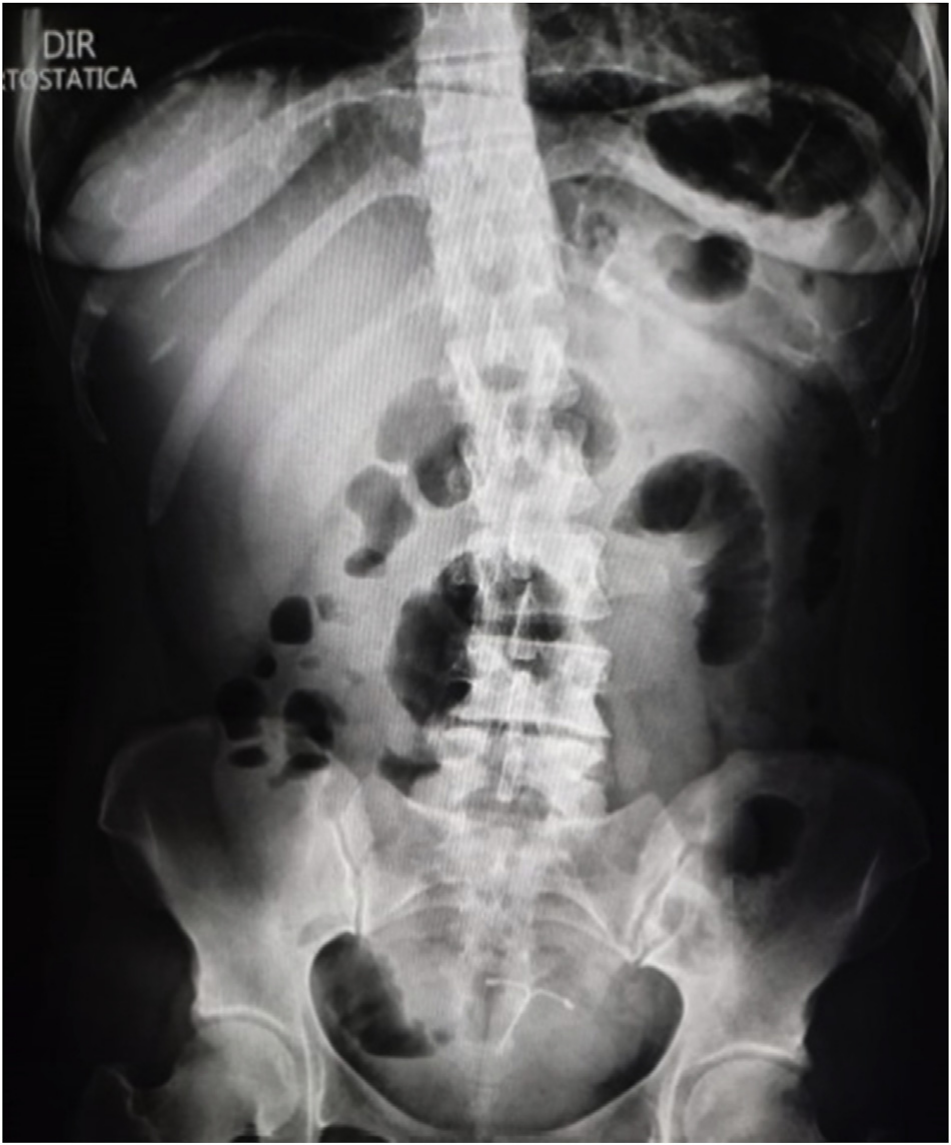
Abdominal Xray showing signs of pneumoperitoneum.
